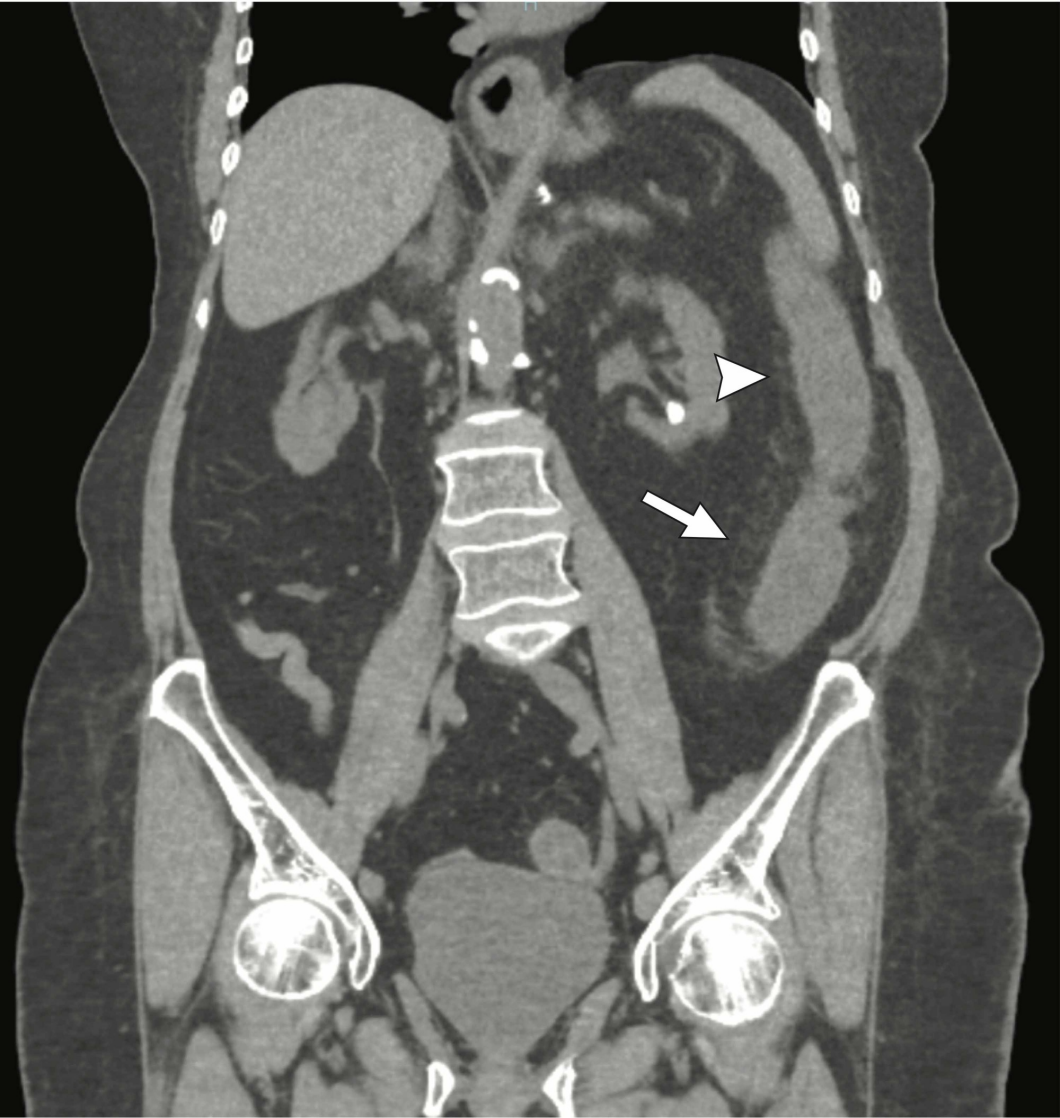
Coronal CT image demonstrating pericolonic inflammatory changes (white arrowhead) and bowel wall thickening (white arrow) of the descending colon.
